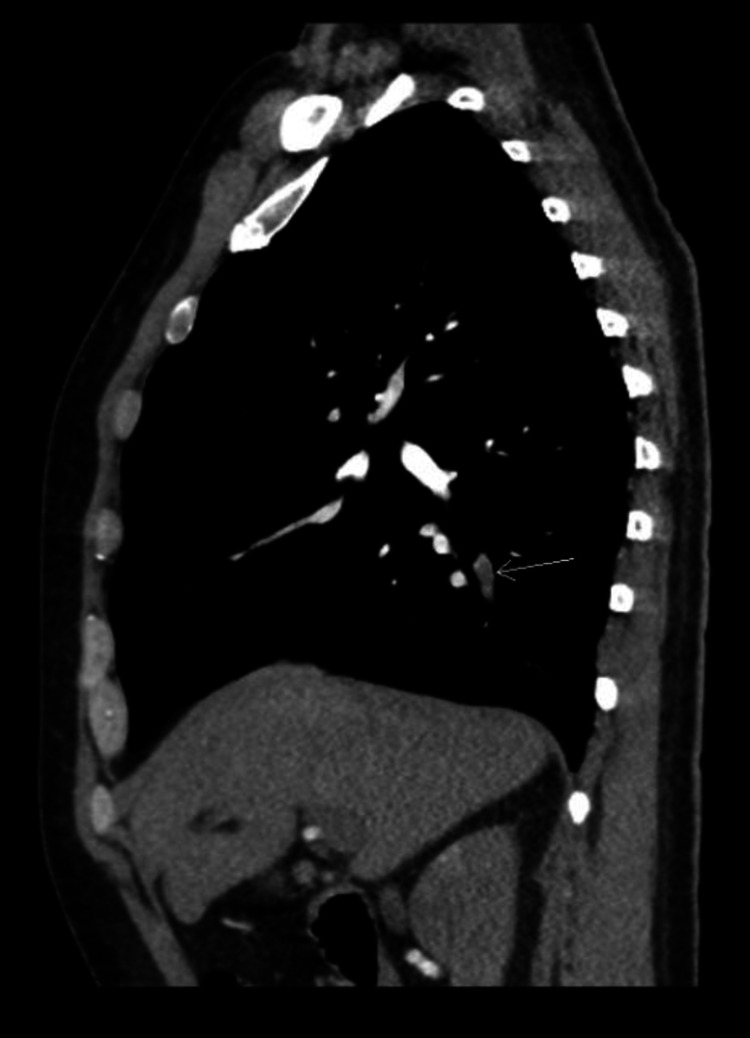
Sagittal view of the contrast-enhanced computed tomography scan of the chest demonstrating pulmonary embolism in the right lower lobe (arrow).
